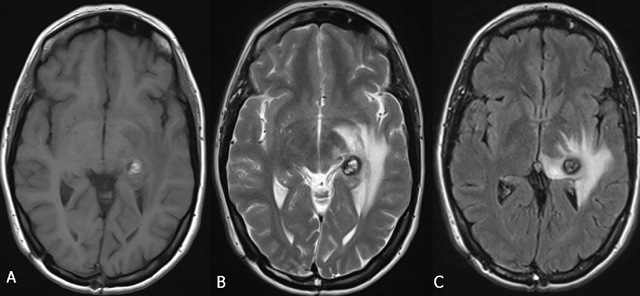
FCCM Type 1 lesion. Axial SE T1-WI (A), TSE T2-WI (B), and FLAIR (C). Hyperintense core on SE T1-WI (A) and SE T2-WI (B) suggests recent hemorrhage. Peripheral oedema is best seen on FLAIR image (C).
